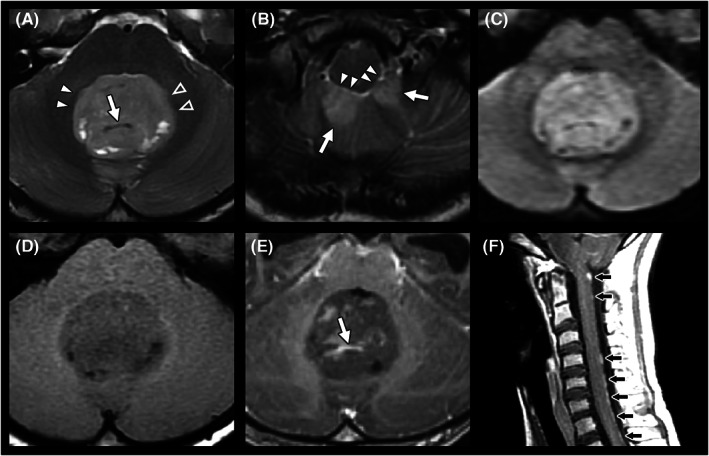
A case of medulloblastoma. This is a 10‐year boy with medulloblastoma. T2WI (A, B) demonstrated a hyperintense mass lesion from bilateral cerebellar tonsils (b, arrows) with upward extension to the fourth ventricle (A). The tumor had clear margins without invasion into the right middle cerebellar peduncle (A, arrowheads) or the brainstem (B, arrowheads). A fuzzy margin indicating local tumor invasion was noted at the left middle cerebellar peduncle (A, open arrowheads), which was later proven by documentation of a left infiltrating tumor in the surgical report. The lesion was hypointense on T1WI (D), showed heterogeneous enhancement after administration of the contrast agent (E), and was hyperintense in relation to the cerebellar white matter on DWI, *b* = 1000 s/mm^2^ (C). A dominant central drainage vein was observed on T2WI (A, arrow) and contrast‐enhanced T1WI (E, arrow). In addition, leptomeningeal seeding was noted on sagittal contrast‐enhanced T1WI (F, black arrows).
